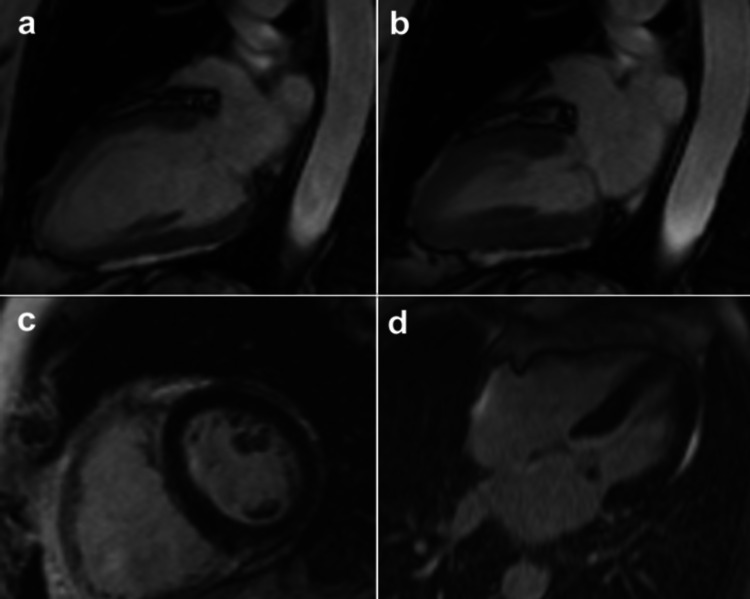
Cardiac magnetic resonance imaging proved normal function LV with no abnormalities in the LV kinetics (a, b) and no LGE (c, d). (a) Cine diastole, (b) cine systole, (c) LGE short axis, (d) LGE four chambers LGE - late gadolinium enhancement
